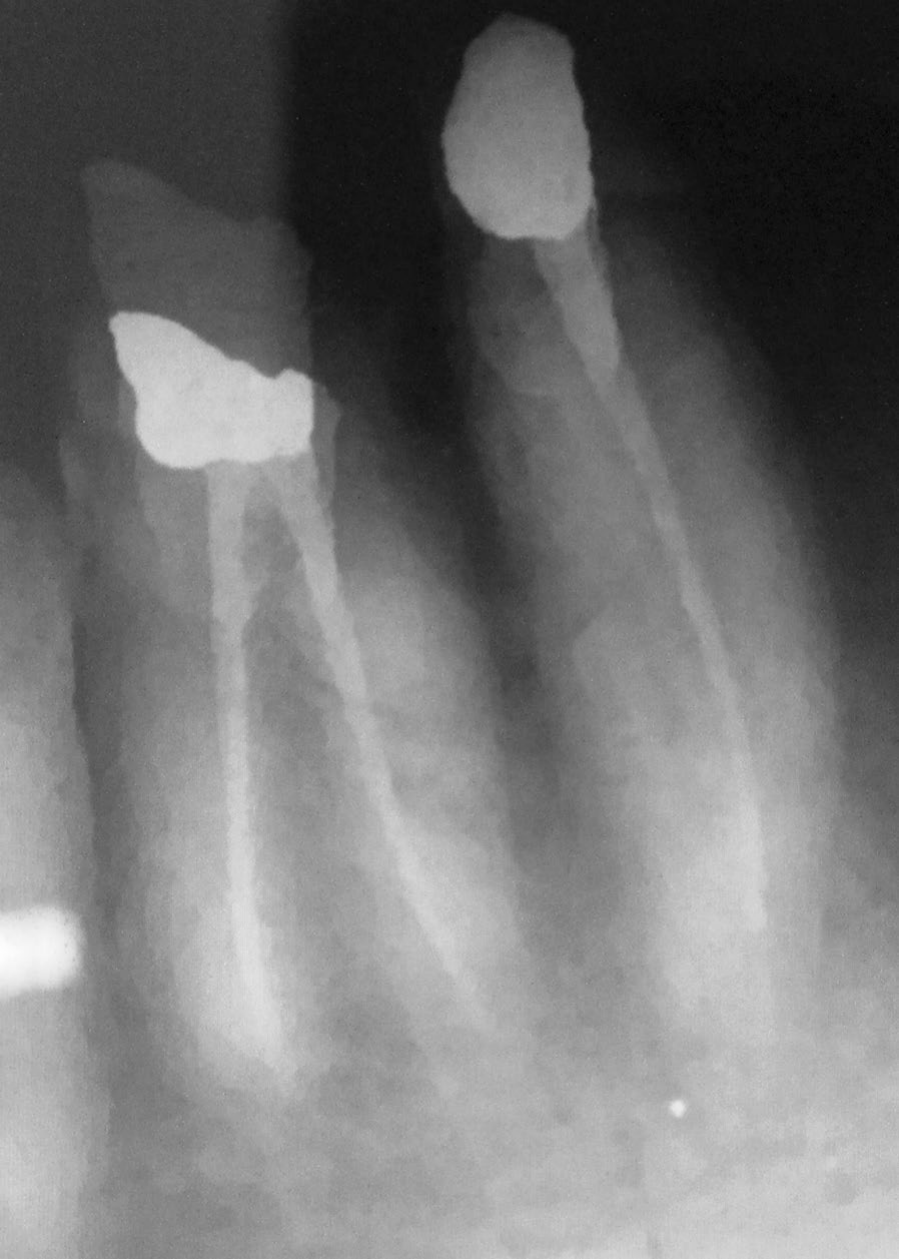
Radiograph showing obturated both canals
